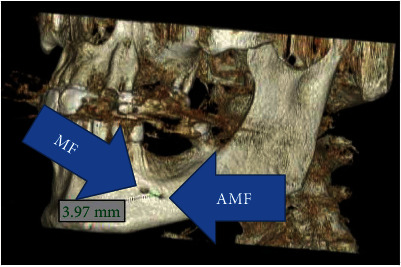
Left AMF at reconstructed CBCT.
